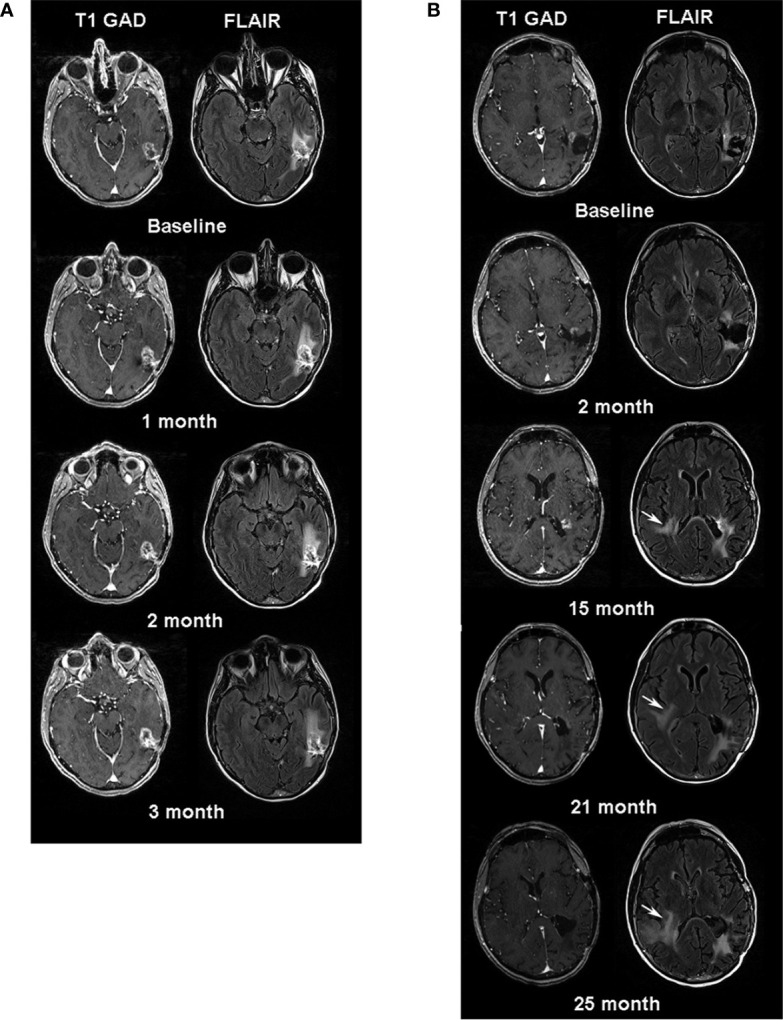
(A) Serial MR images one week before (baseline) and three months after CAR T-EGFRvIII infusion. Left sided images are axial post contrast T1 weighted images and right sided images are axial FLAIR images. Note, minimal increase in size of the enhancing lesion in the left temporal lobe at follow-up time-points. (B) Serial MRI examinations after the patient’s second surgery. The baseline is one month after her second surgery. White arrows at 15, 21- and 25-month follow-up periods indicate the increasing signal abnormality in the right periventricular region on FLAIR images.
